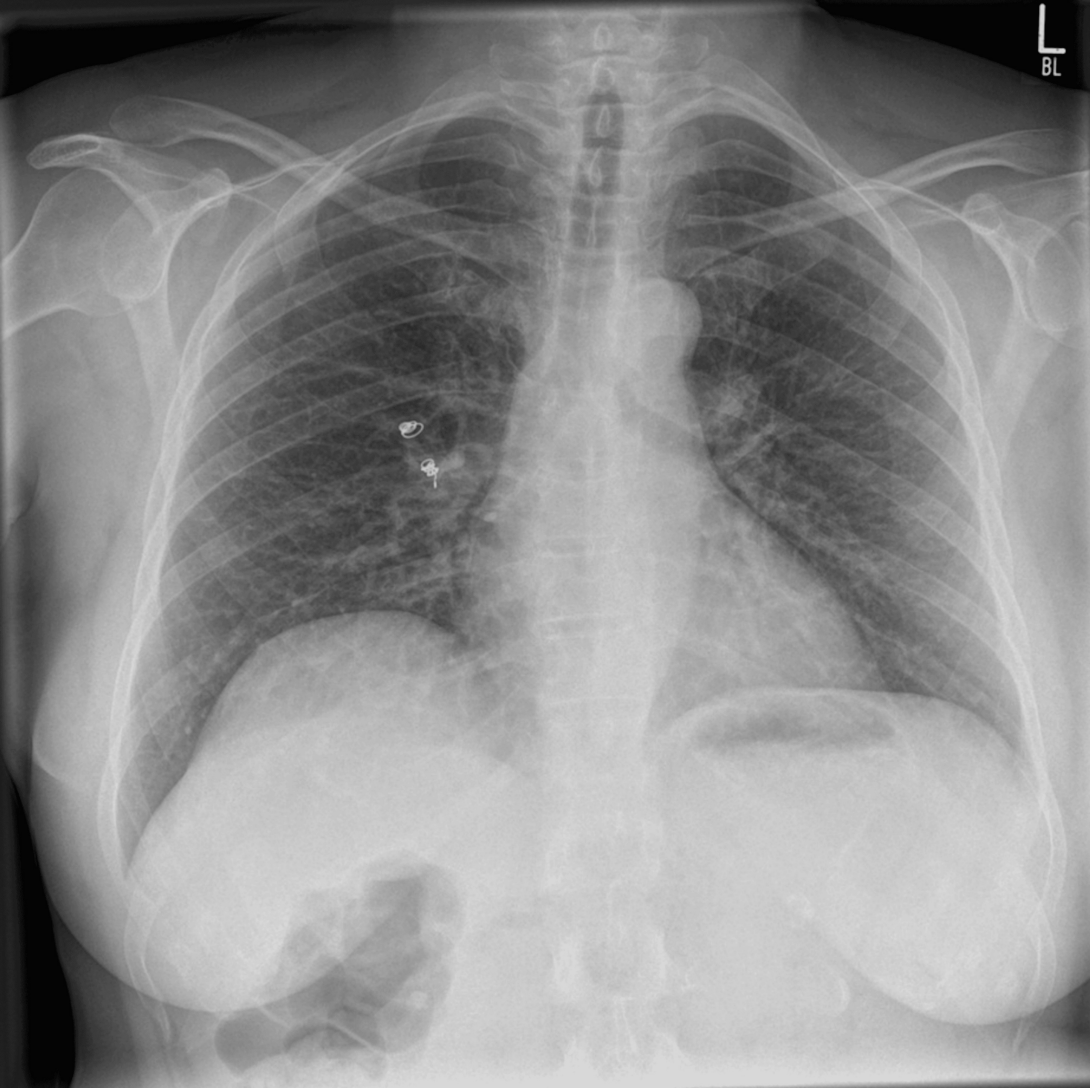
Postinterventional chest X-ray showing coil placement.
